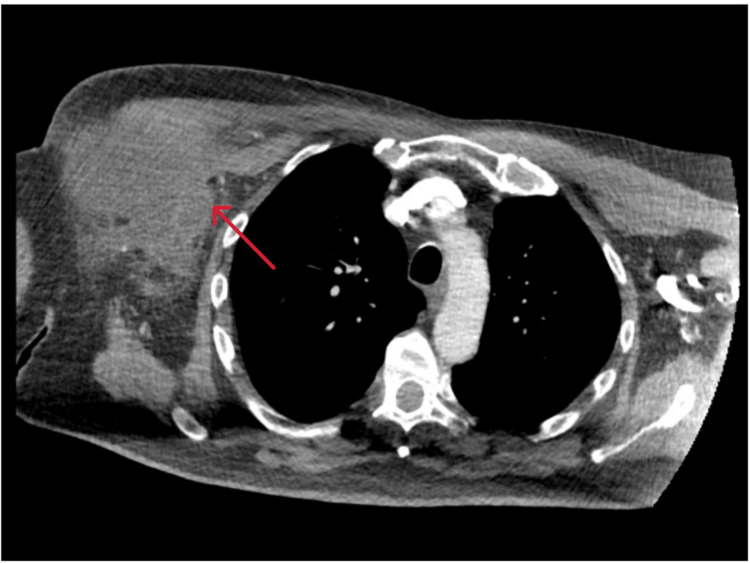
Computed tomography image showing a collection in the subcutaneous tissue of the right axilla and right breast
